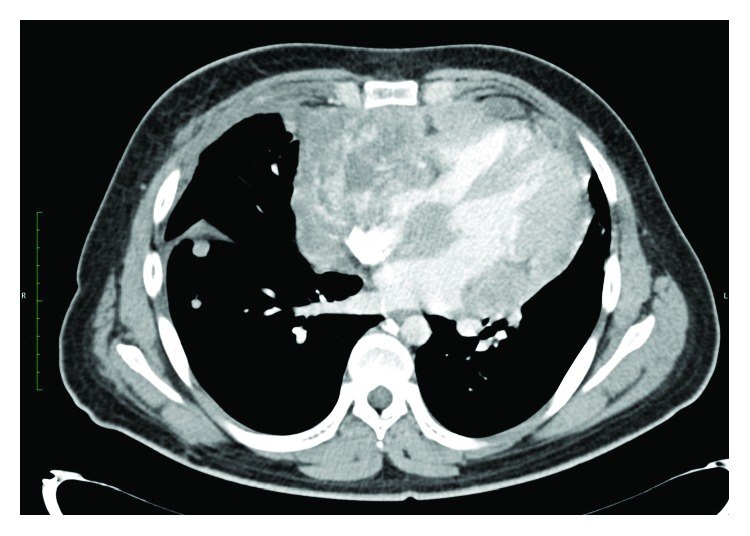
Chest CT scan with IV contrast showing a hyperdense mass within the pericardial space, with infiltration into the myocardium, displacing and compressing adjacent structures.
